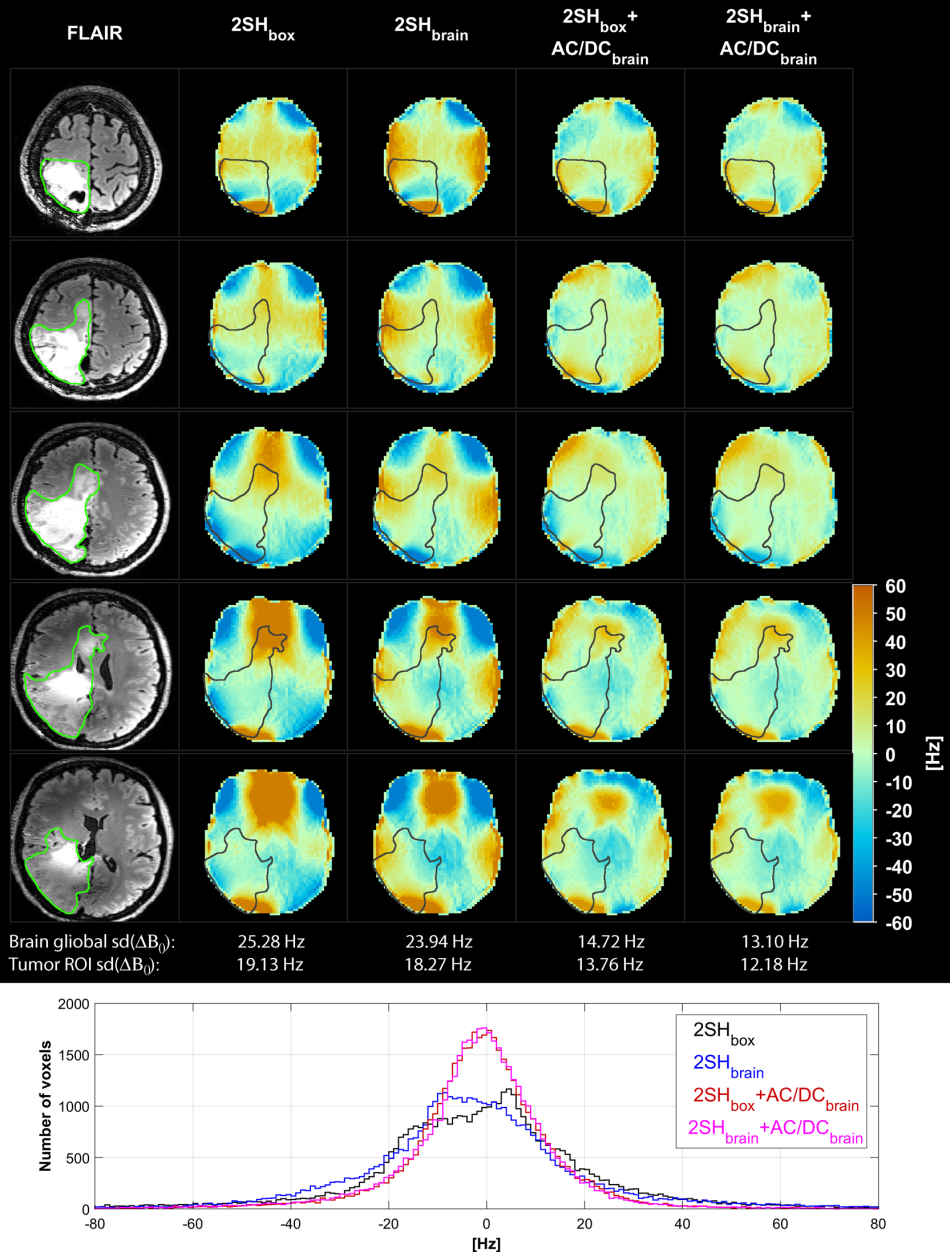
Comparison of B_0_ fieldmaps in mutant IDH1 glioma patient under four shimming conditions 2SH_box_, 2SH_brain_, 2SH_box_ + AC/DC_brain_, and 2SH_brain_ + AC/DC_brain_. Five representative slices are shown through the shimmed whole-brain slab with the tumor ROI contours. Standard deviation of B_0_ distributions is given below for the entire slab and the tumor. Histograms of B_0_ distribution over the entire brain slab are shown overlaid at the bottom for all the shimming conditions. FLAIR anatomical image is shown on the left most column.
